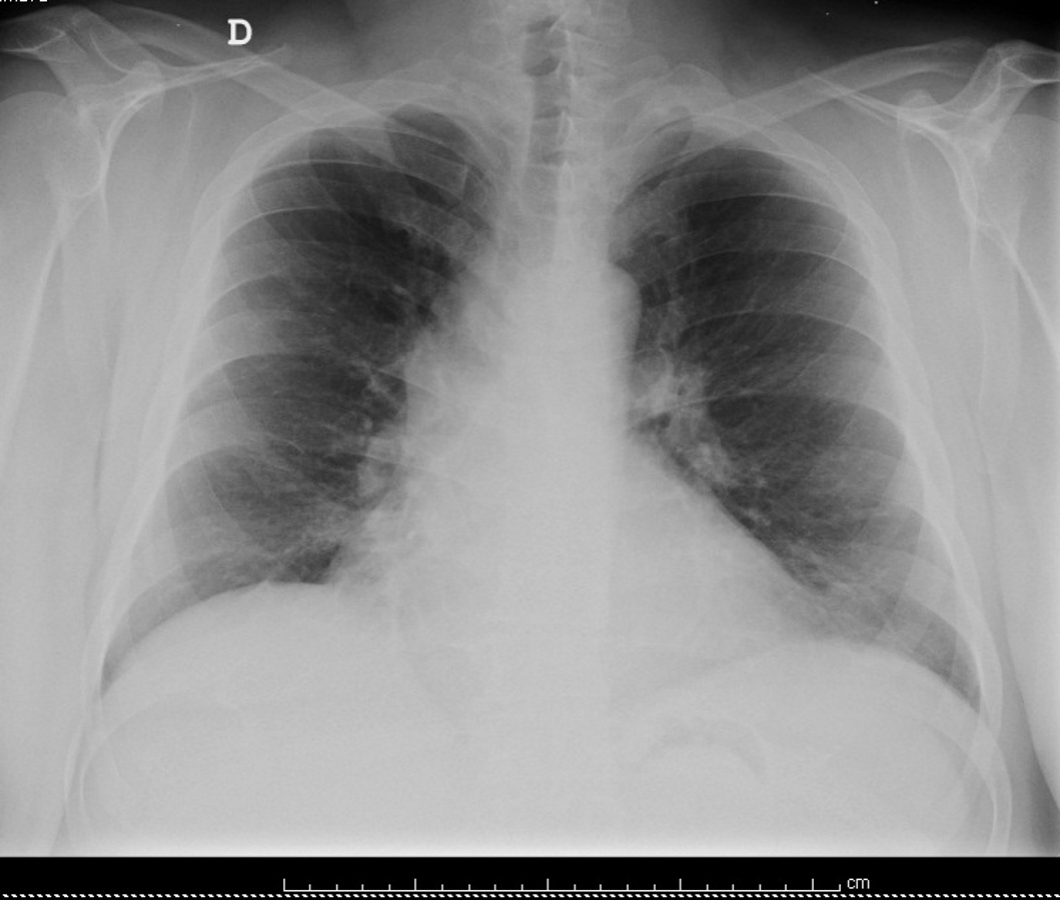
Chest radiography during follow-up. Complete radiological resolution is observed.
